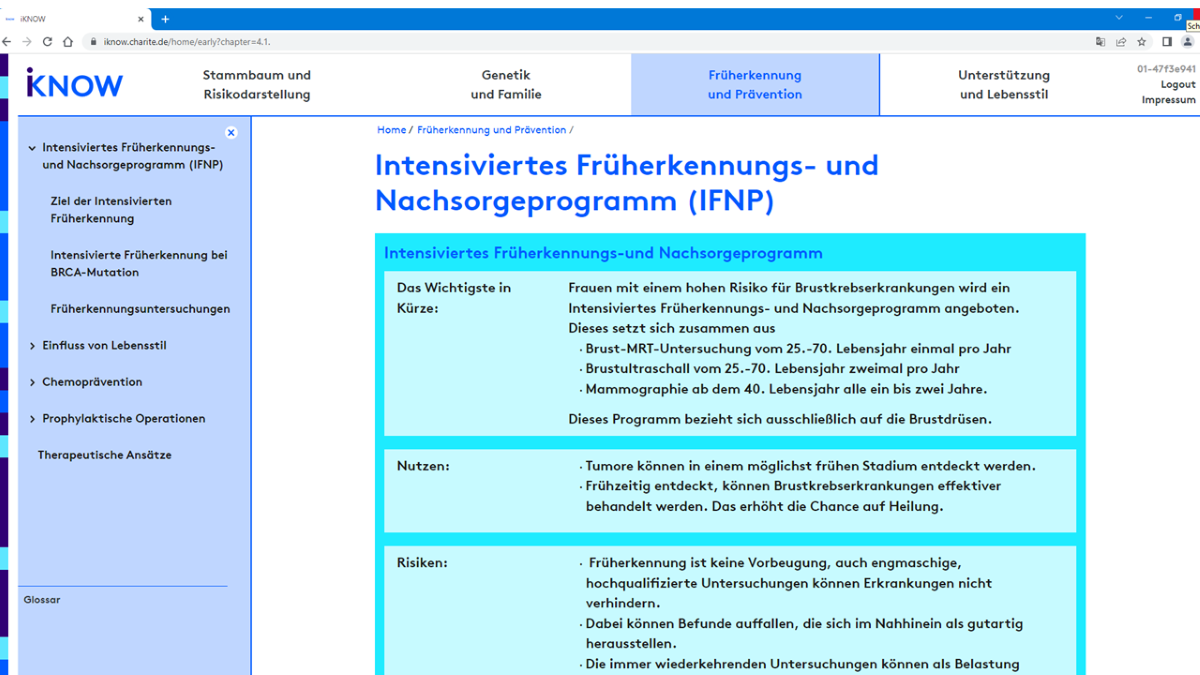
iKNOW counseling tool. To allow patients to look up information after counseling, a concise, text-based summary of counseling contents is provided in addition to the visuals presented during counseling, conveying a takeaway message and a verbal summary of benefits (eg, early tumor detection) and risks (eg, false alarms). BRCA: breast cancer gene; MRT: MRI (magnetic resonance imaging).
